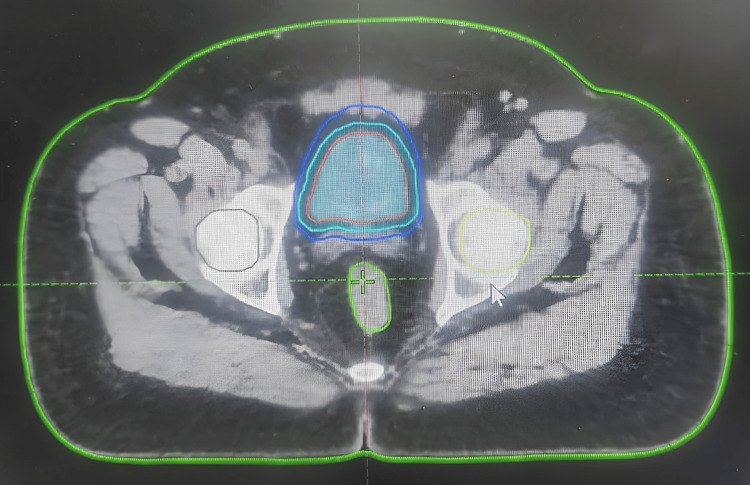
Planning computed tomogram with CTV, small PTV, and large PTV and OARs red: CTV; cyan blue: small PTV; dark blue: large PTV; yellow: left femoral head; brown: right femoral head; green: rectum CTV: clinical target volume; PTV: planning target volume; OAR: organs-at-risk (rectum and femoral heads)
